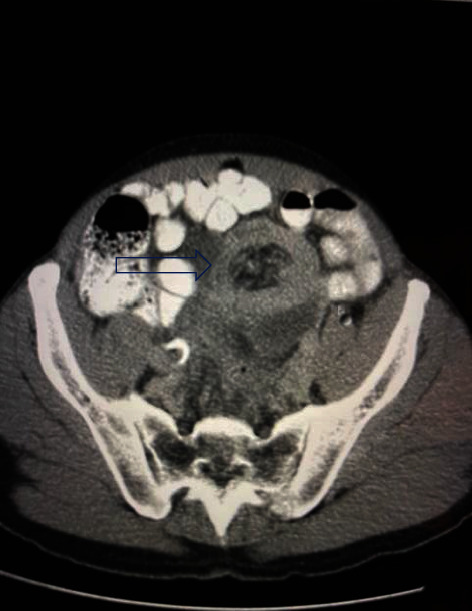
Axial view of the abdominal CT scan showing intussusception.
